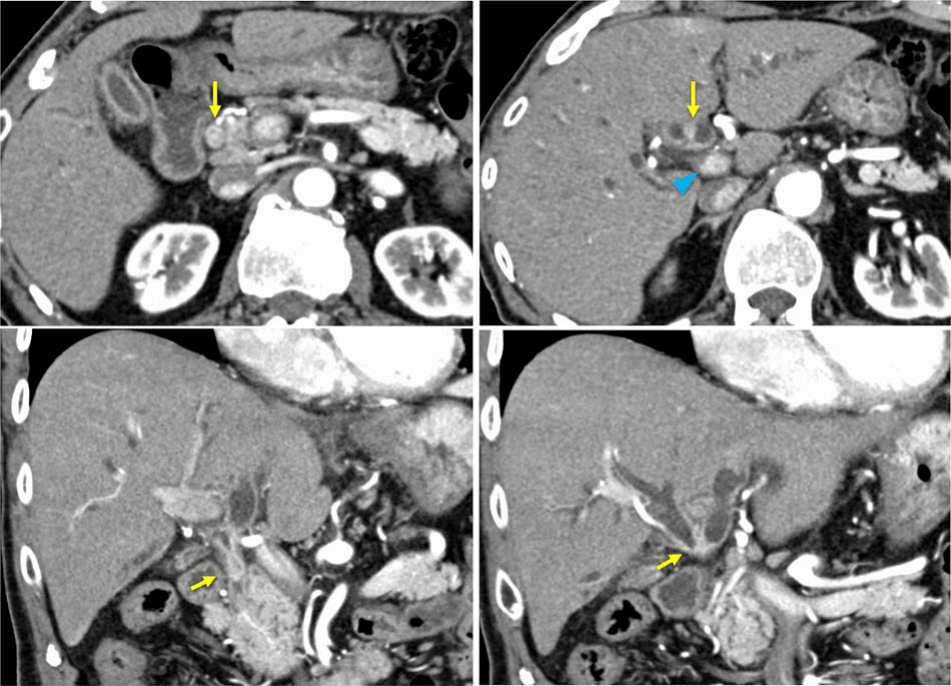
Preoperative contrast-enhanced computed tomography. Hilar bile duct thickening (arrows) and intrahepatic bile duct dilation are observed. The portal vein (arrowhead) is close to the thickened hilar bile duct.
